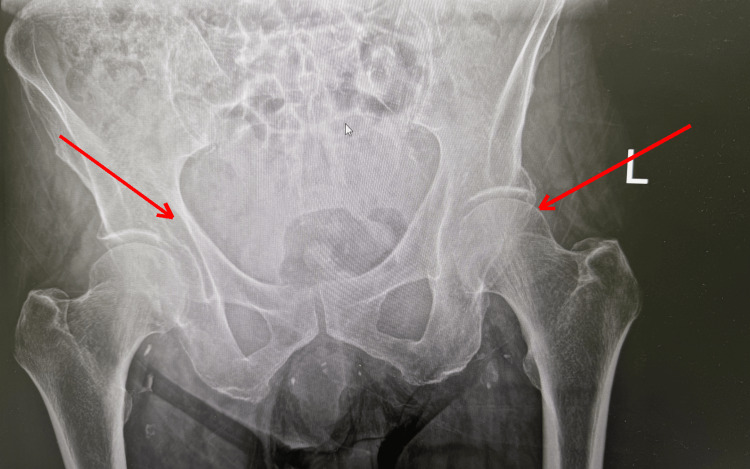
Initial plain pelvic and bilateral hip radiograph Initial plain pelvic and bilateral hip radiograph obtained during orthopedic assessment. Straight digital arrows indicate the clinically symptomatic bilateral hip regions. No acute fracture, dislocation, or clear structural abnormality was identified to account for the severity of symptoms.
